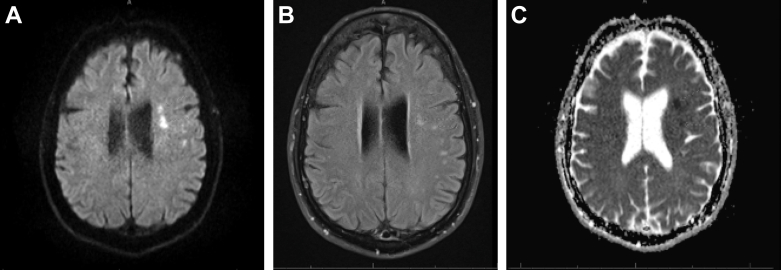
Magnetic Resonance Imaging (A) Magnetic resonance imaging (MRI) diffusion-weighted imaging. (B) MRI fluid-attenuation inversion recovery. (C) MRI apparent diffusion coefficient. MRI revealed multiple areas of cerebral infarction including the left operculum, insular cortex, and left internal capsule with subtle hemorrhagic conversion.
